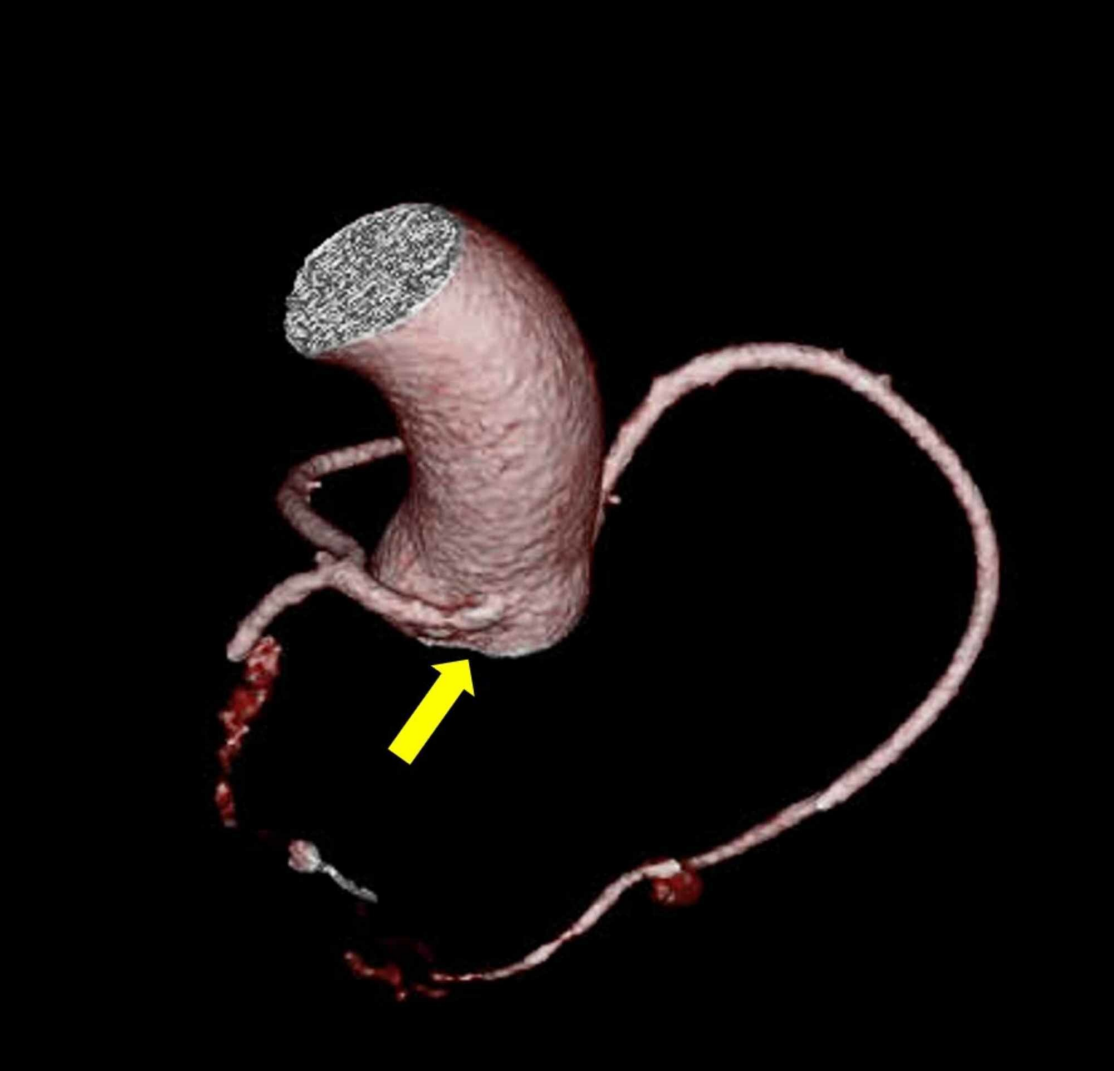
Tridimensional CT reconstruction showing LCANCC Tridimensional computed tomography reconstruction with leftward rotation showing the posterior origin of the LCA (arrow). CC: computed tomography; LCANCC: left coronary artery from the non-coronary cusp; LCA: left coronary artery
